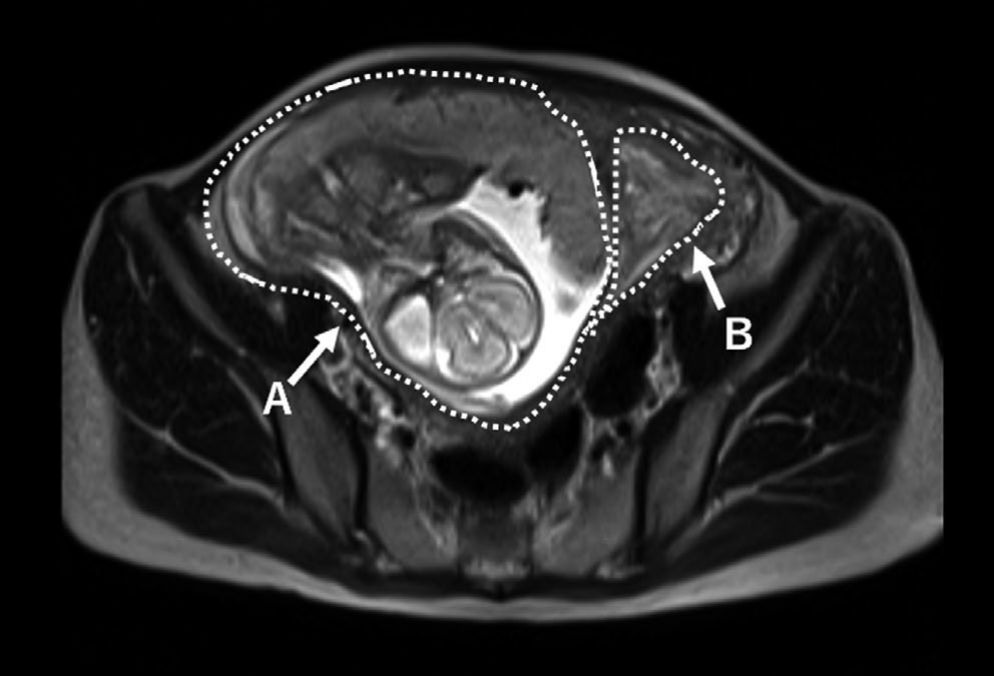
Magnetic resonance imaging (MRI) of septate uterus at 21 wk and 5 d of gestation. A, A T2‐weighted MRI reveals a fetus in the right side of the uterus with no signs of hematoma or thickened placenta. B, The left side of the uterus contains a hematoma
